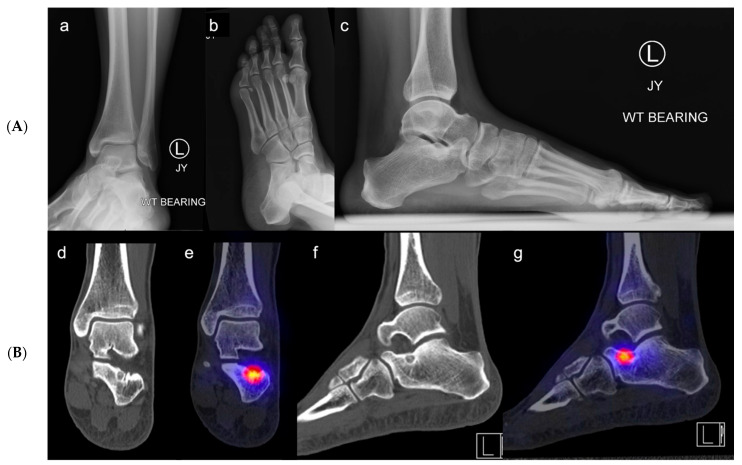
(A,B). Osteoid osteoma of the calcaneus at the floor of the sinus tarsi. Case of a 26-year-old female patient with left sinus tarsi pain. (a) ap, (b) oblique, (c) lateral X-rays (A) of the left foot and ankle. On the lateral X-ray view (c) there are sclerotic changes in the calcaneus at the area of the calcaneal sulcus. Further imaging with SPECT-CT was performed (d–g). (d,e) show coronal views with a cystic and sclerotic intraosseous lesion with a central nidus. (f,g) show sagittal views with the nidus on the floor of the sinus tarsi. On the SPECT-CT imaging (B) (e,g) there is increased uptake at the site of the lesion. The bony lesion is highly suspicious for osteoid osteoma.
